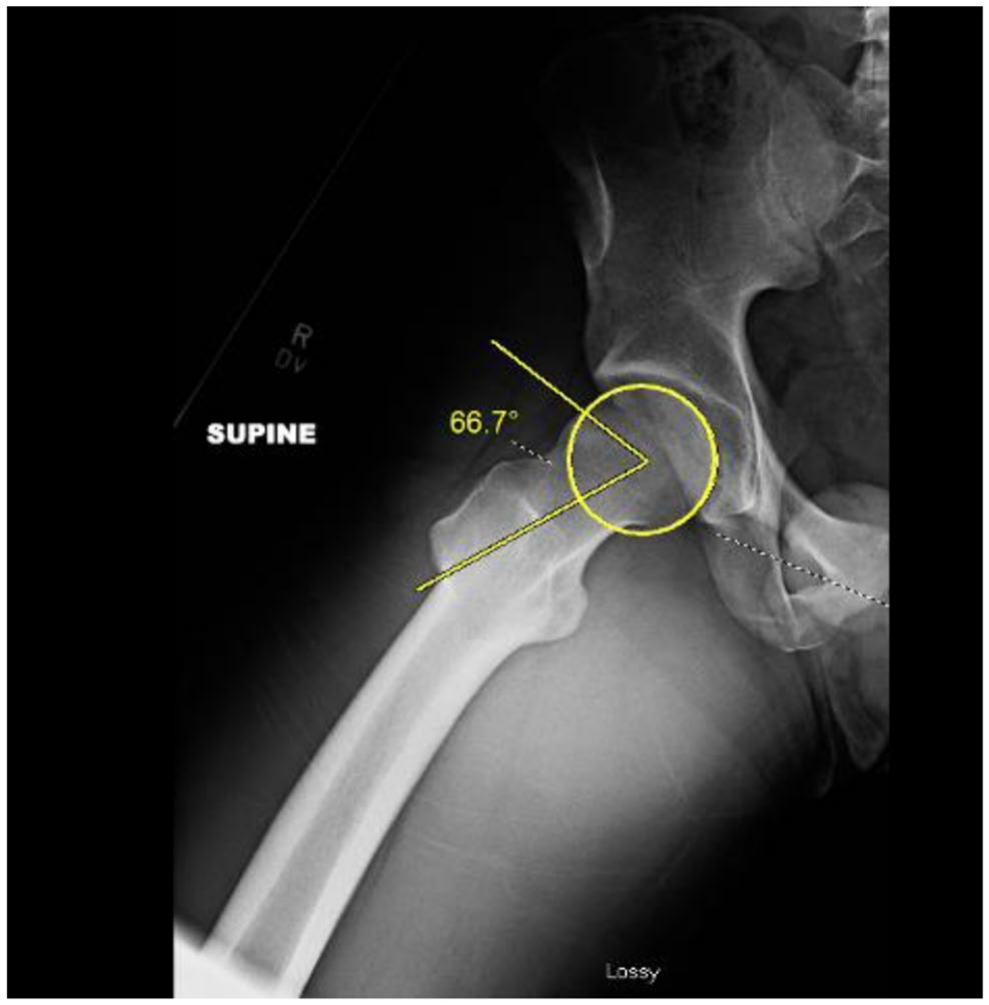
Alpha angle measured on 45^o^ Dunn Lateral radiograph
